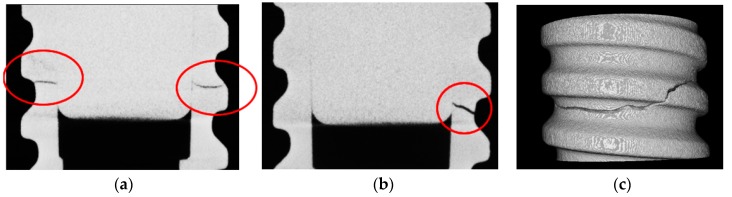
Single slices (a,b) and 3D view (c) of implant and dental abutment assemblies made of Ti_40_Zr_10_Cu_36_Pd_14_ BMG after fatigue test and obtained by X-ray tomography.
